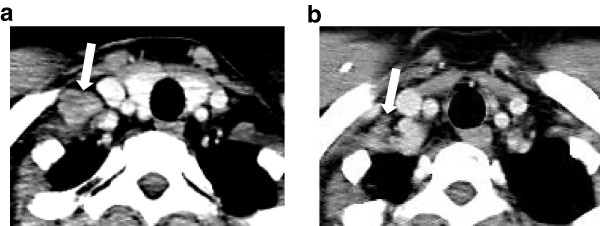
Cervical computed tomography demonstrates a swollen supraclavicular lymph node. The size decreased from 20mm (a: arrow) to 5mm (b: arrow) after FOLFOX.
